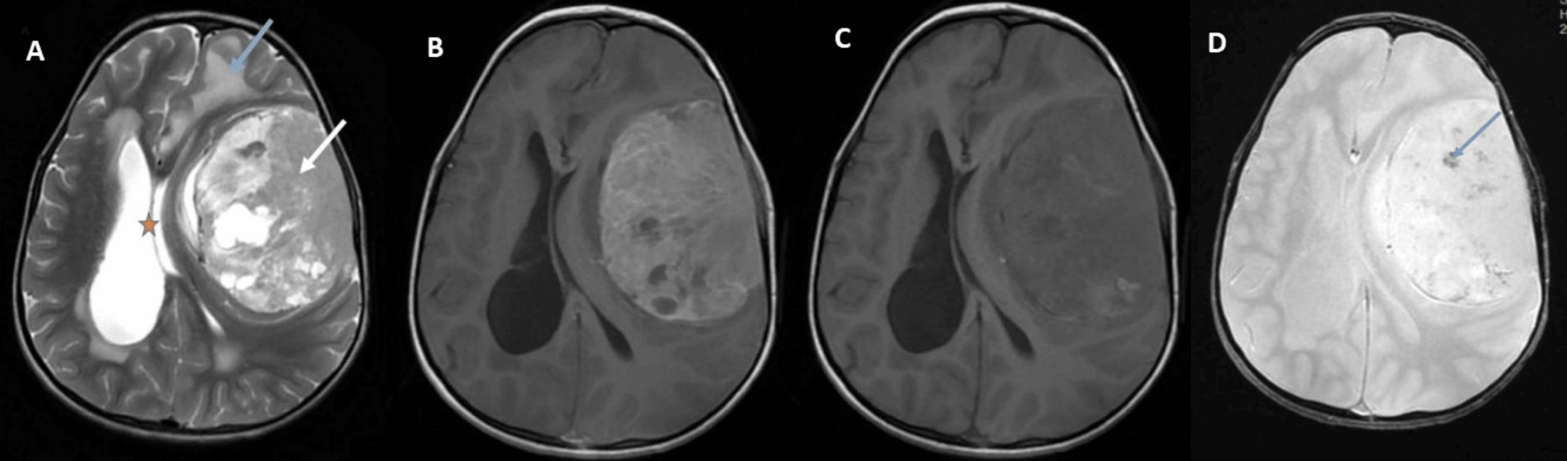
Pretherapeutic brain magnetic resonance imaging (MRI). (A) T2 axial image showing a large fronto-parieto-temporal meningioma (white arrow) in hypersignal with heterogeneous aspect with mass effect on the midline structures (red star) and peri-lesional edema (blue arrow). (B) T1 axial image showing a large fronto-parieto-temporal meningioma with iso-intense signal and heterogeneous aspect. (C) T1 axial image post contrast injection showing a large fronto-parieto-temporal meningioma with intense heterogeneous enhancement. (D) Susceptibility axial pretherapeutic image showing microcalcifications (blue arrow).
